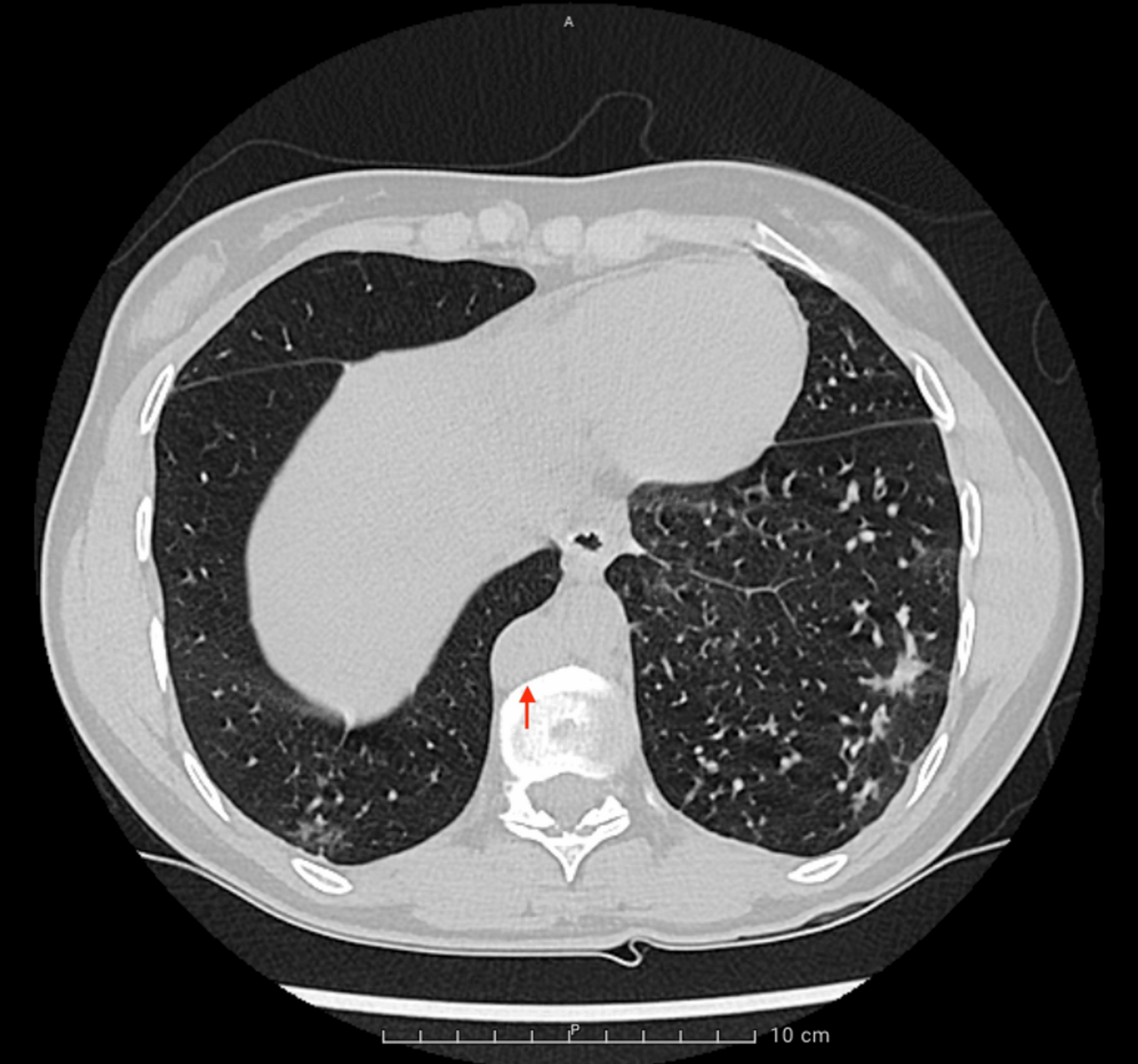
Chest CT demonstrating azygos continuation of the inferior vena cava (red arrow) and diffuse bronchiolitis pattern
